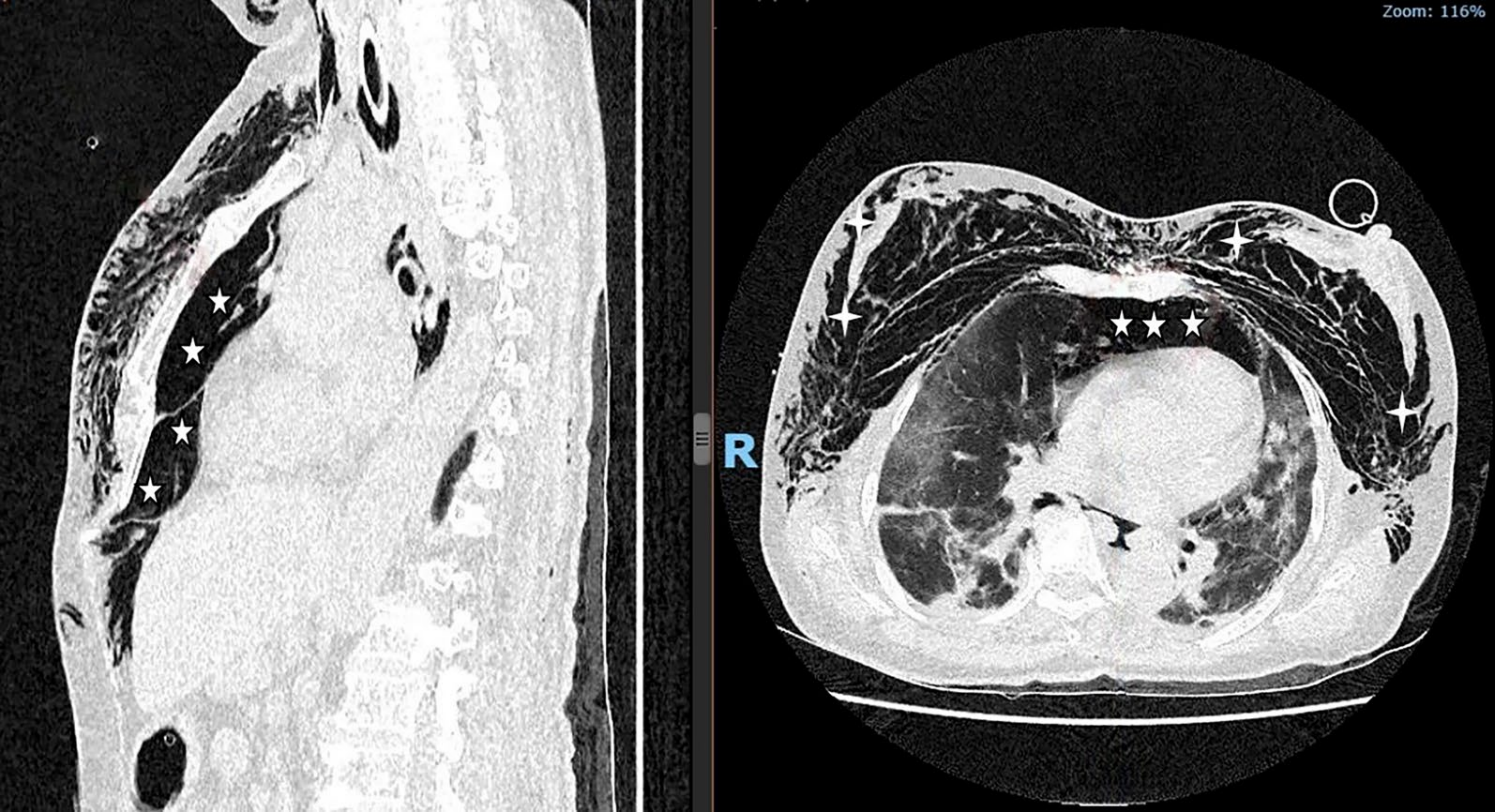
Thorax computed tomography of tension pneumomediastinum before decompression. Left panel: An important accumulation of air in the upper mediastinum extending downward to the preperitoneal cavity (*five-point stars*). Right panel: In addition to the pneumomediastinum, computed tomography showed diffuse subcutaneous emphysema (*four-point stars*)
